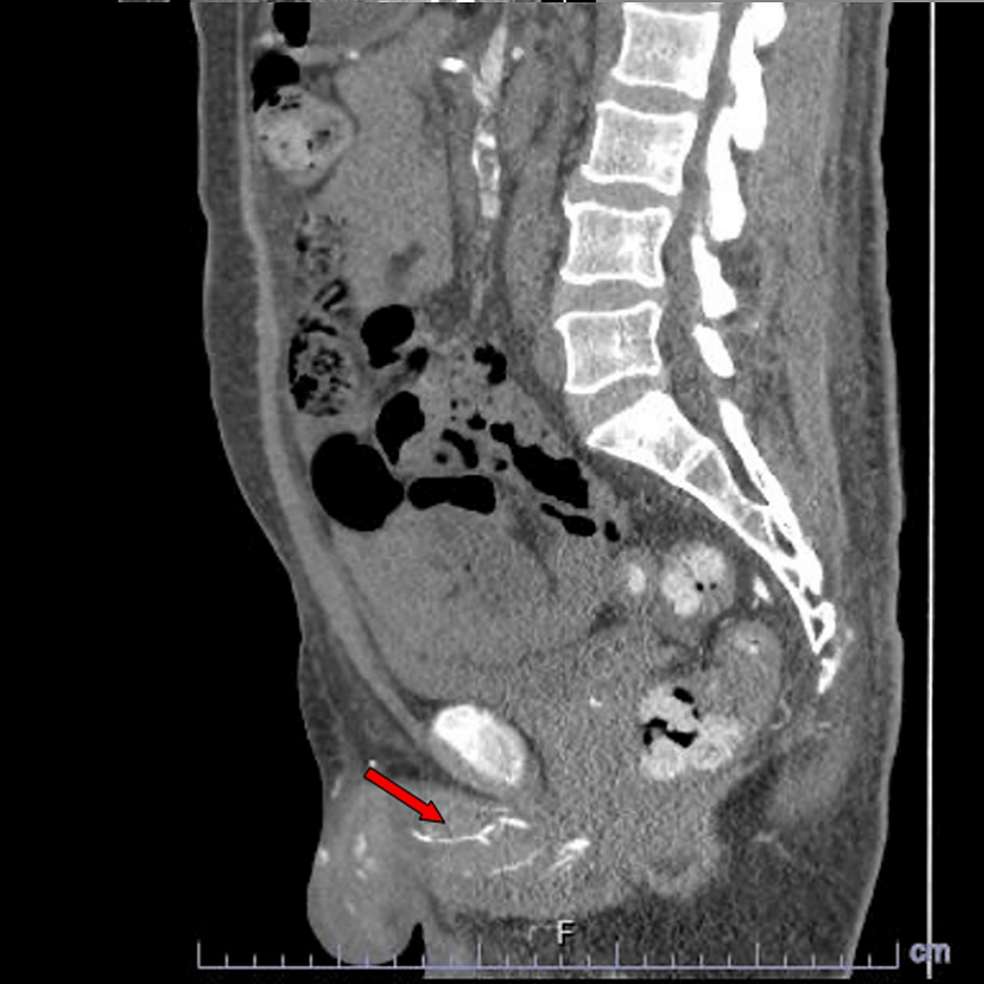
Pelvis CT scan showing diffuse calcification of penile vessels
